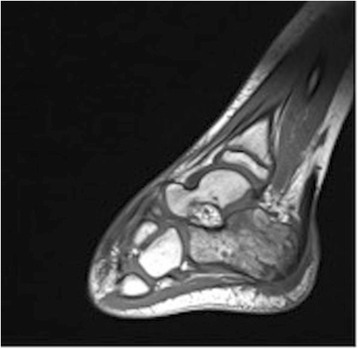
Sagittal magnetic resonance imaging scan of the left foot revealing osteomyelitis of the calcaneum
